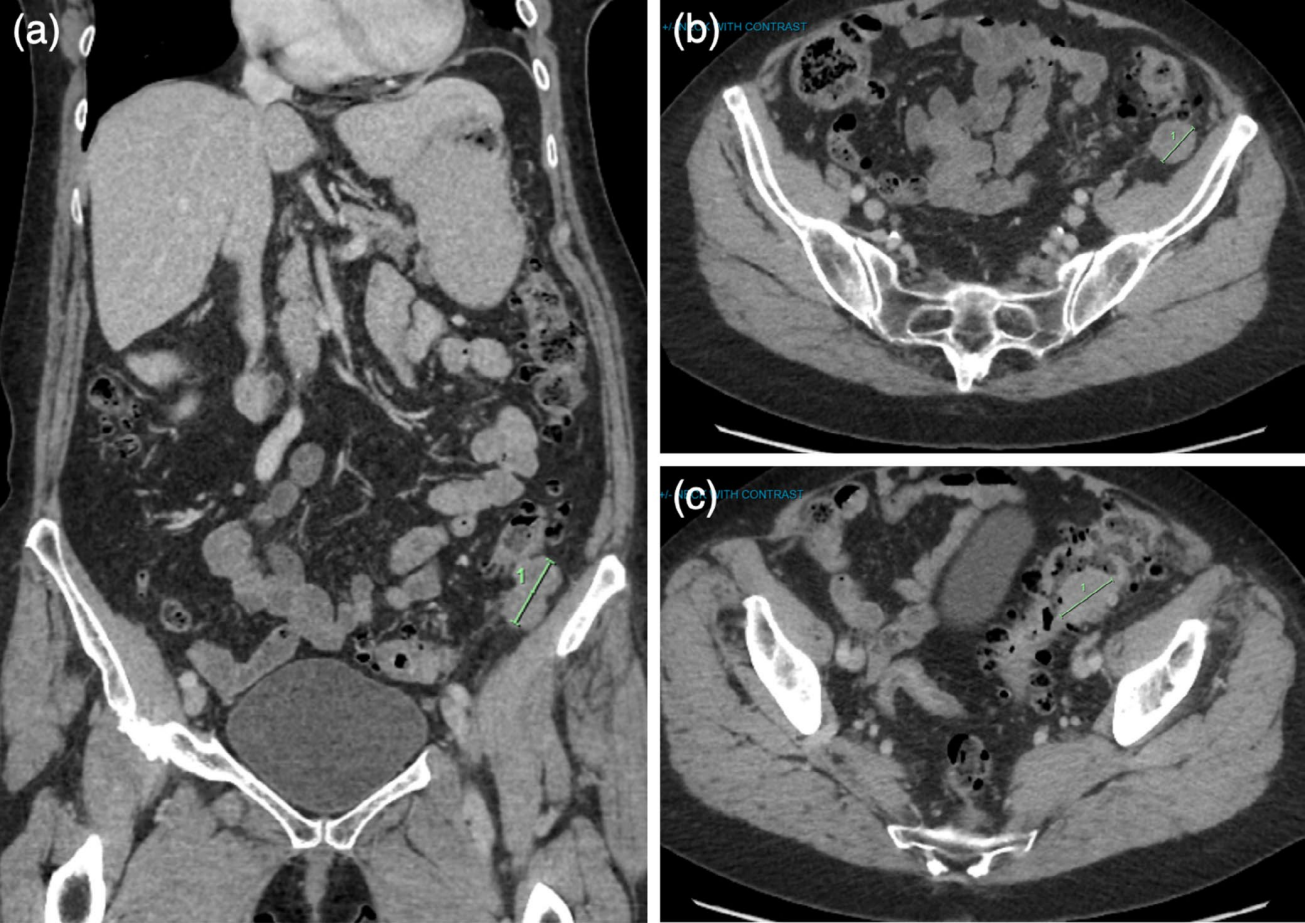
CT images. (a) A bilobed solid lesion was seen within the pericolic fat posteriorly abutting the sigmoid colon. The lesion extended over a length of 38 mm in the coronal plane. (b) The solid lesion measured 25 mm in the axial plane. (c) Focal ovoid area of soft tissue thickening within the sigmoid colon at the left iliac fossa. The lesion involved the colonic wall and measured approximately 35 mm.
